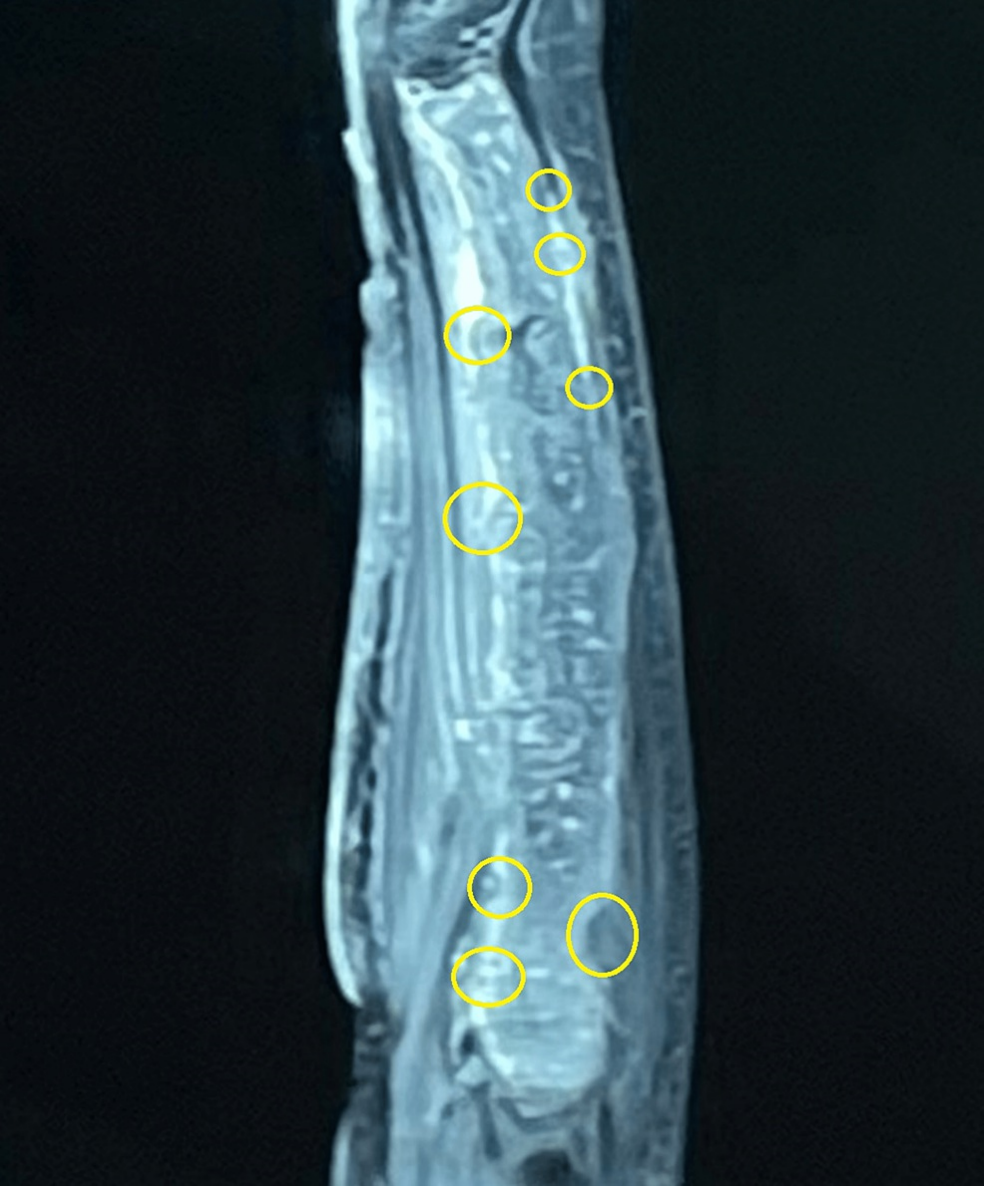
MRI of the right forearm showing cortical thickening and an irregular outline to the entire length of the radius. Yellow circles: Multiple ill-defined lytic areas on the inner aspect of the radius.
